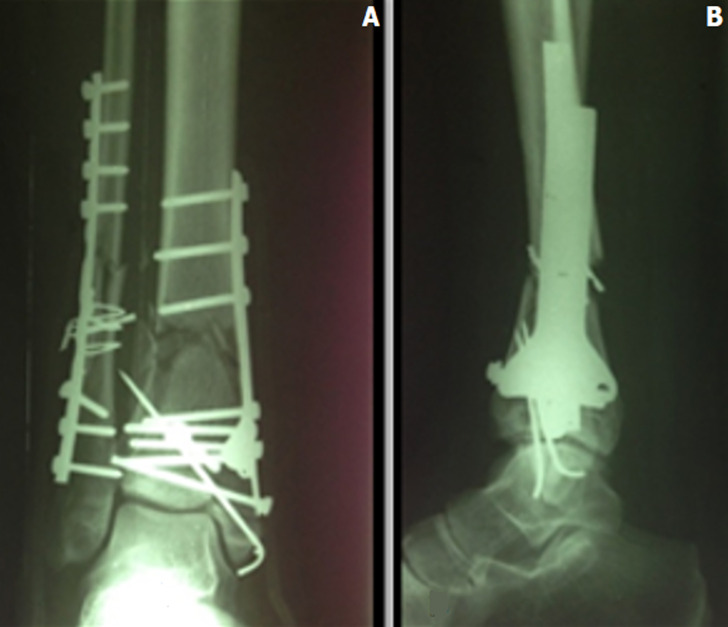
radiographie de la cheville face (A) et profil (B) montrant une synthèse par plaque vissé en trèfle et embrochage avec synthèse de la fibula par plaque vissé 1/3 de tube et cerclage
